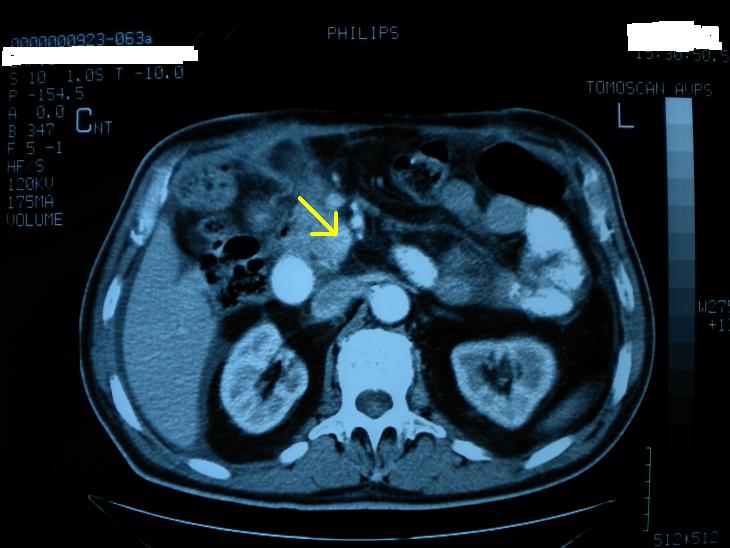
Contrast enhanced CT scan depicting the 1.5 cm solid mass in the uncinate process of the pancreas (arrow).
